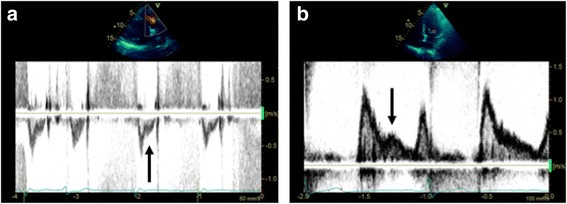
Additional information provided by Doppler flow pattern analysis. a Flying ‘W’ sign with pulsed wave Doppler analysis of right ventricle outflow tract: a highly specific sign of pulmonary hypertension. b L-wave on mitral valve inflow: seen in relatively bradycardic patients with normal hearts or in a pathological setting in those with elevated left ventricle preload and moderate diastolic dysfunction
